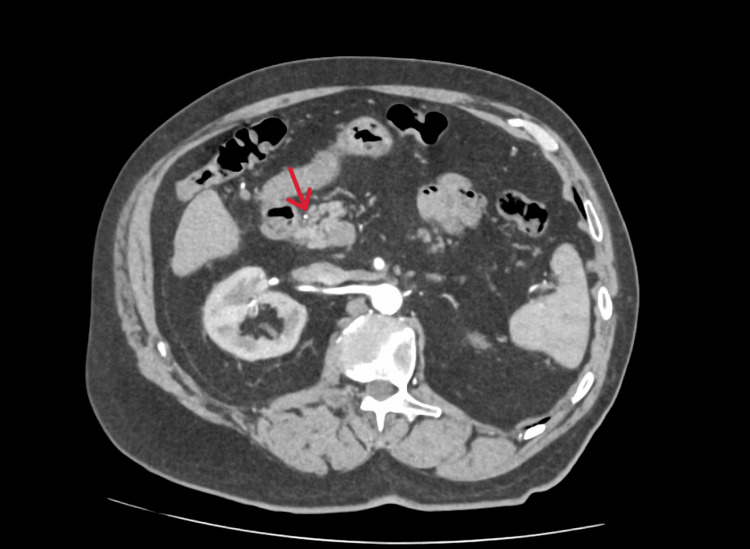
Computed Tomography (CT) Pancreas with the section of the head of pancreas showing no pathology The red arrow indicates the head of the pancreas.
